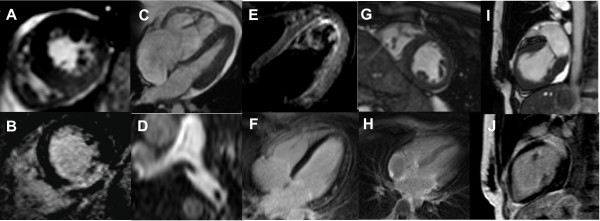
A+B) 63 year-old patient with inferior wall AMI, T2-edema and hypo-kinesia on short- axis SSFP-images (A) and concomitant LGE (B). C+D) 29 year-old patient with excessively dilated right chambers and systolic dysfunction on SSFP-images (C) revealing thrombi in the proximal left pulmonary artery (D) on pulmonary angiography. On echocardiography the patient showed only insufficient image quality. E+F) 45 year-old patient with intermitting fever and edema at the LV lateral wall on T2-four chamber images (E) without wall motion abnormalities but clear patchy, infarct-atypical LGE images confirming the diagnosis of myocarditis (F). G+H) 32 year-old patient with slightly elevated cTnT levels, moderately reduced ejection fraction (EF = 43%), symmetric myocardial hypertrophy (G) and diffuse LGE patterns (H) suspicious of cardiac amyloidosis. I+J) 62 year-old woman with signs of mid-ventricular ballooning (I) without edema or LGE (J) was classified as tako-tsubo CMP.
